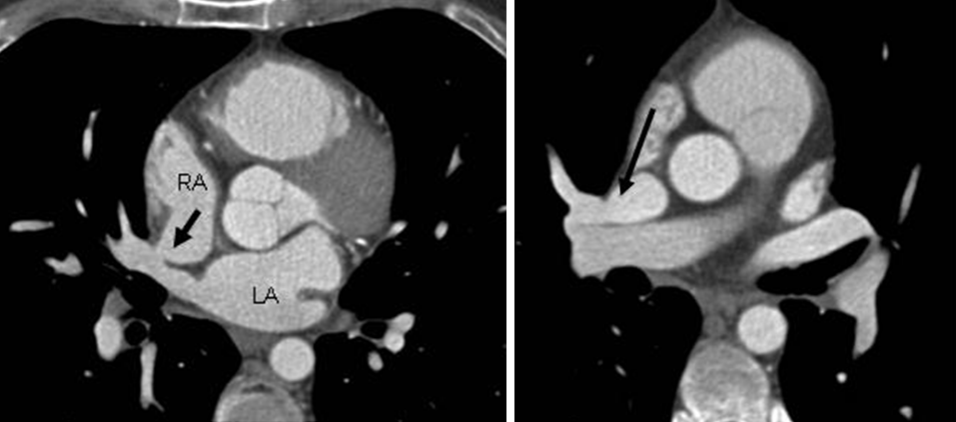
A superior sinus venosus atrial septal defect (ASD) and partial anomalous pulmonary venous return (PAPVR) on the right side. A hypertensive male patient with dilatation of the right ventricle documented with echocardiography. Multidetector computed tomography angiography showed a sinus venosus ASD (a) and PAPVR from the right upper lobe to the superior vena cava (b). RA = right atrium, LA = left atrium.
